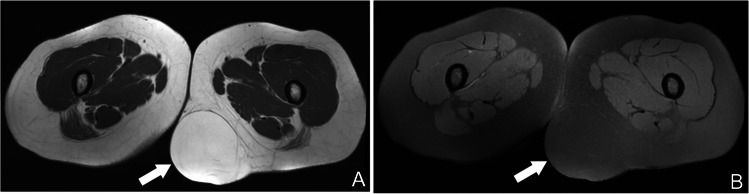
Axial MR images in a 49-year-old woman demonstrate an encapsulated superficial lipoma. (A) T1-weighted image and (B) fat-suppressed T2-weighted image show a well-circumscribed, homogeneously hyperintense mass within the subcutaneous fat (arrows), identical to subcutaneous fat signal in all sequences. A fine, low-signal-intensity fibrous capsule surrounds the lesion, confirming its encapsulated nature
